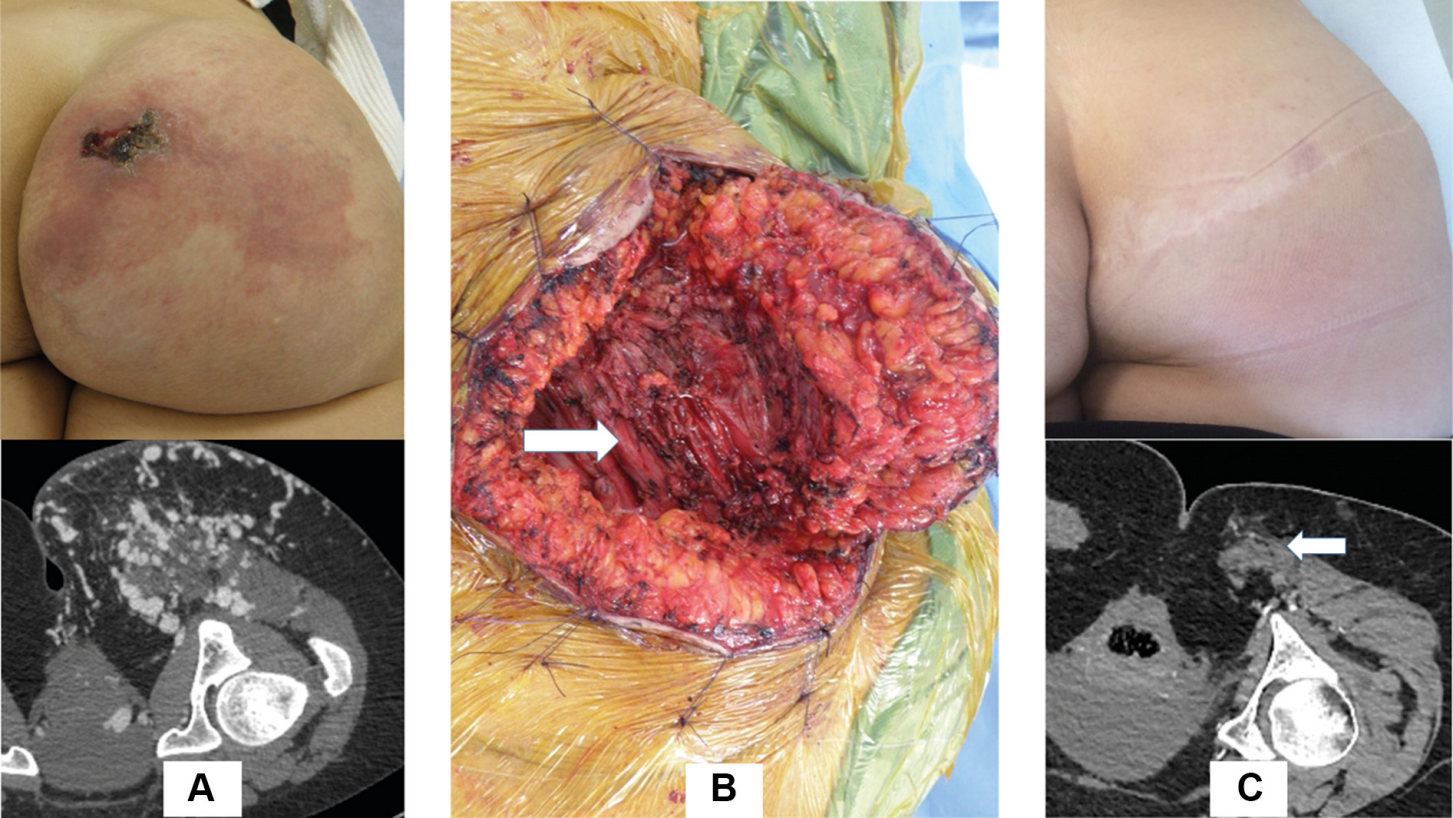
A, Preoperative photograph and computed tomography (CT) scan of an arteriovenous malformation (AVM). B, Intraoperative photograph of an AVM (*arrow*). C, Postoperative photograph and CT scan showing the repaired AVM (*arrow*).
